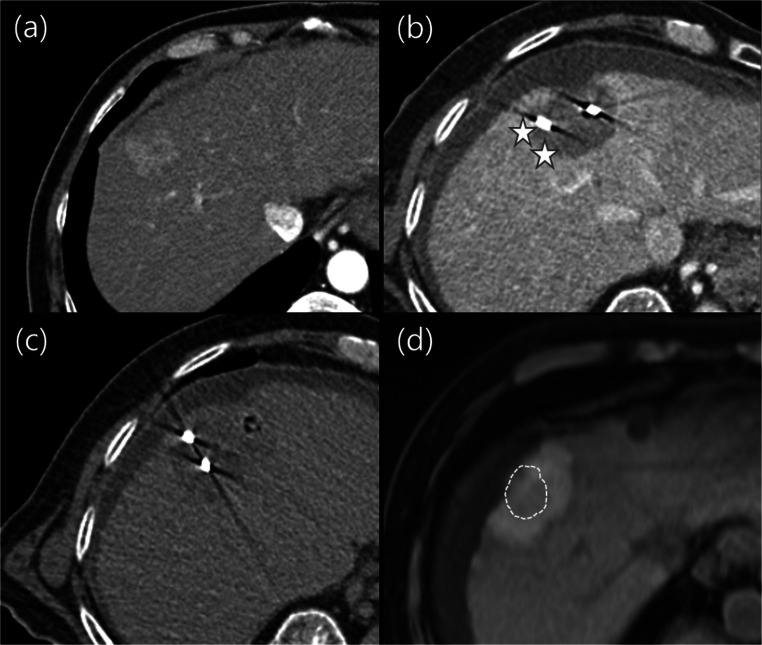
Patient with hepatocellular carcinoma (HCC) treated with microwave ablation (MWA). Pre-treatment arterial phase CT axial image (a) shows a hypervascular HCC at segment VIII of the liver. After expected ablation completion, ablative margin was assessed to be insufficient at the right side of tumor (stars) on intra-procedural CT images (b). Immediate additional ablation was performed by repositioning of MWA probes (c) to cover the site of insufficient margin. On post-MWA MRI, pre T1-weighted axial image (d) demonstrates that sufficient margin more than 5 mm is obtained around the tumor surface (dashed line) even at the right side of the tumor. Note that artificial ascites was introduced in this case due to subcapsular location of tumor and to minimize thermal injury to the diaphragm
